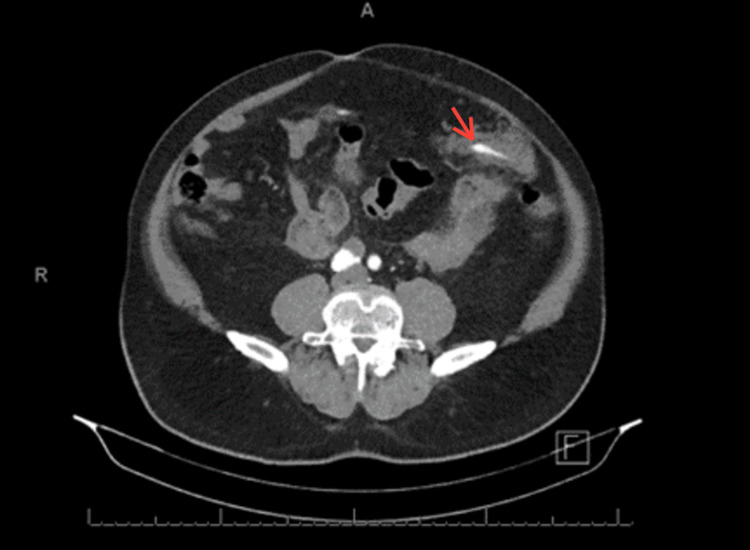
Enhanced CT scan axial view showing focal dilation of omental artery.
